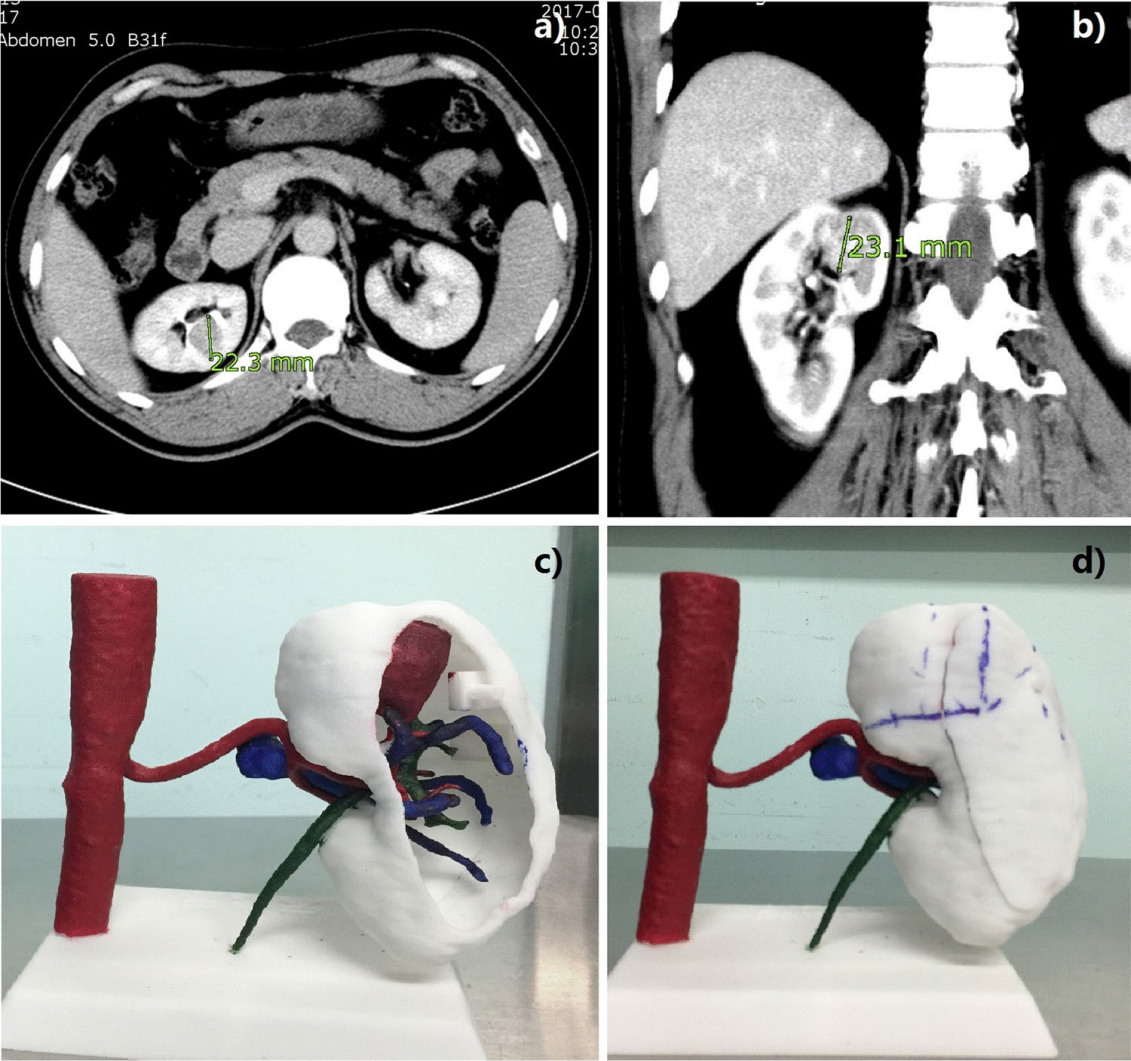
Computed tomography images (a,b) and 3D kidney model (c,d). The endophytic mass markers are labeled on the surface of the models after preoperative planning (d). The renal artery is an important external anatomical landmark.
